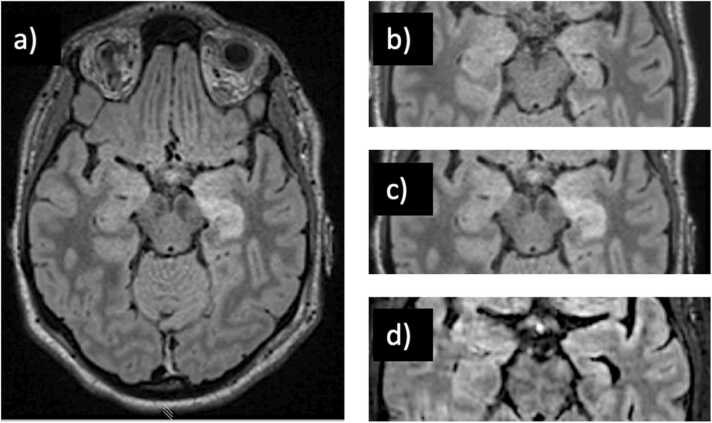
A patient with anti-NMDA receptor encephalitis (a) Whole-brain FLAIR image demonstrating hyperintense signal change and enlargement of the left hippocampus. This finding was not seen on initial MRI (b). (c) Cropped FLAIR image of MRI performed on day 5 for comparative purposes. (d) Improvement of findings upon initiation of corticosteroids and plasmapheresis with development of left hippocampus atrophy on long-term follow-up.
